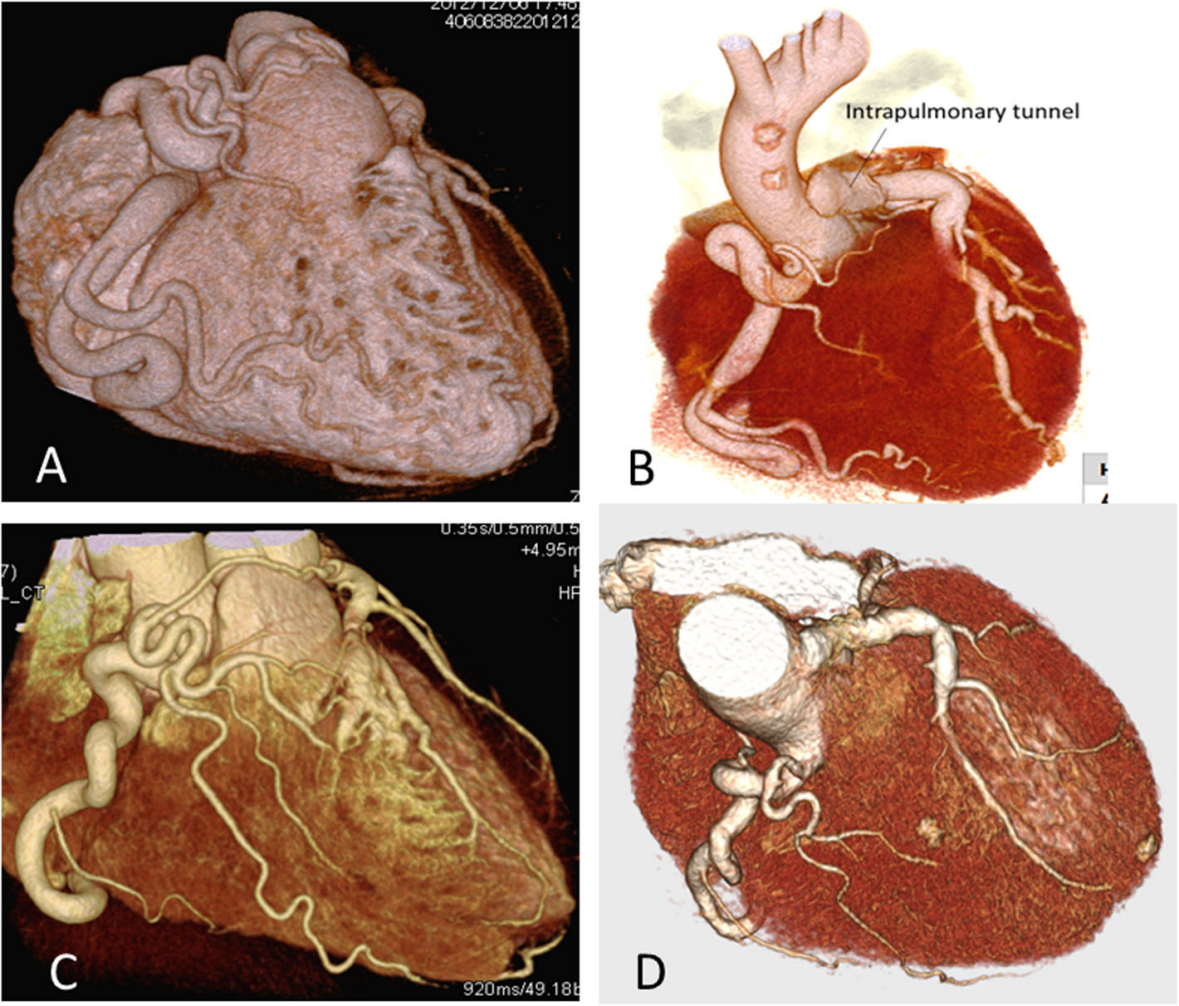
Pre- and post-operative 3D-computed tomography. a. Preoperative 3-D CT in Case 1. b. Postoperative 3-D CT in Case 1. c. Preoperative 3-D CT in Case 2. d. Postoperative 3-D CT in Case 2. Preoperative 3-D CT revealed a large RCA with extensive collateral filling of the left coronary vascular territory, and the left main trunk connected directly to the pulmonary trunk. Postoperative 3D-CT confirmed diminished size of the collateral arteries and an anatomically corrected LCA by means of an intrapulmonary baffle tunnel
